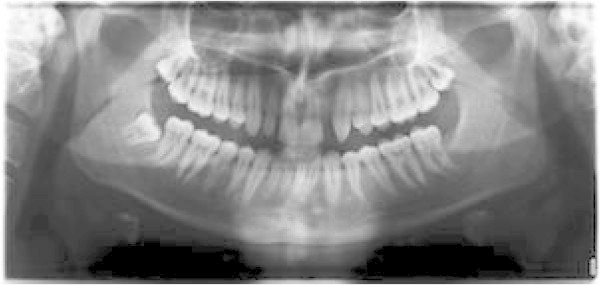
Pre-treatment panoramic X-ray.
